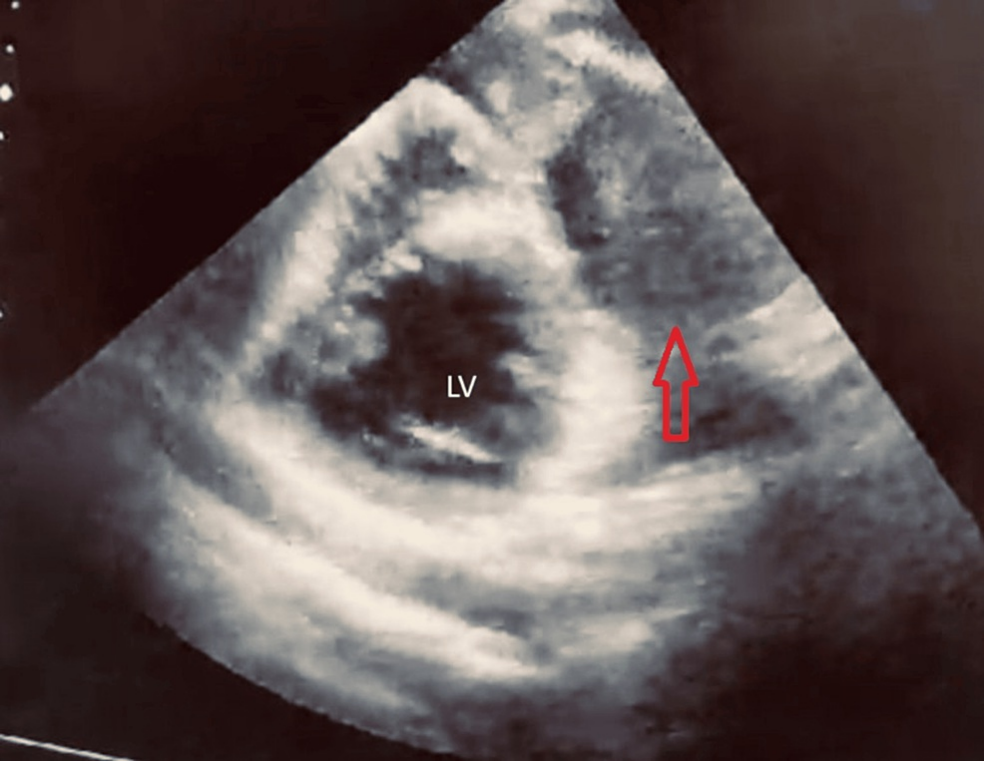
Transthoracic echocardiogram showing the pseudoaneurysm cavity (pointed by the red arrow) communicating with the left ventricular cavity.
